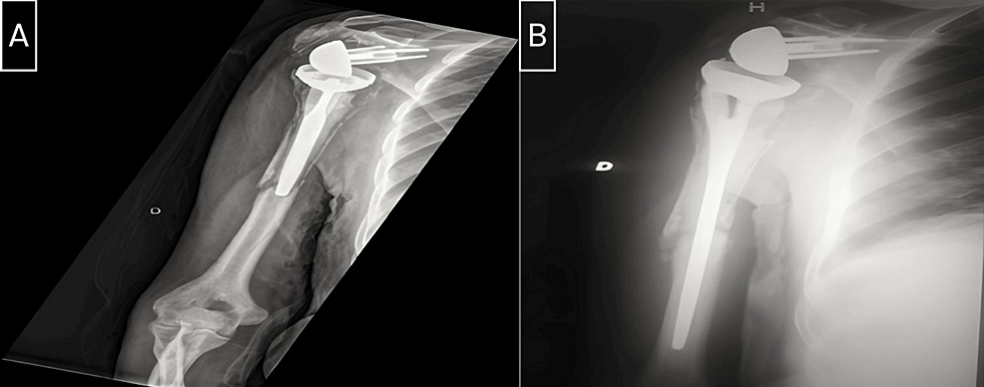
A: Frontal radiograph of the right shoulder shows a periprosthetic fracture of region 3. B: Frontal radiograph of the right shoulder shows the evolution after eight months post-revision.
